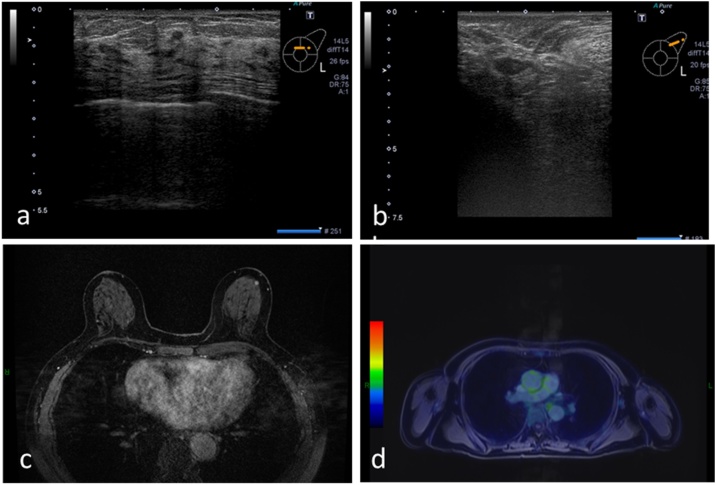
Imaging findings obtained at our institution. (a) Breast ultrasonography revealed a 3- × 2-mm mass with a clear boundary in the upper outer region of the left breast. (b) Breast ultrasonography revealed that the double structure of the left axillary lymph node disappeared and it shrunk compared to the previous findings; the lymph node now measured 15 × 7 mm. (c) Positron emission tomography/magnetic resonance imaging (PET-MRI) revealed a point-like enhancement effect with gadolinium (Gd), indicating fluorodeoxyglucose (FDG) accumulation. (d) PET-MRI revealed that FDG accumulation in the left axillary lymph node was conspicuous compared to the contralateral side.
